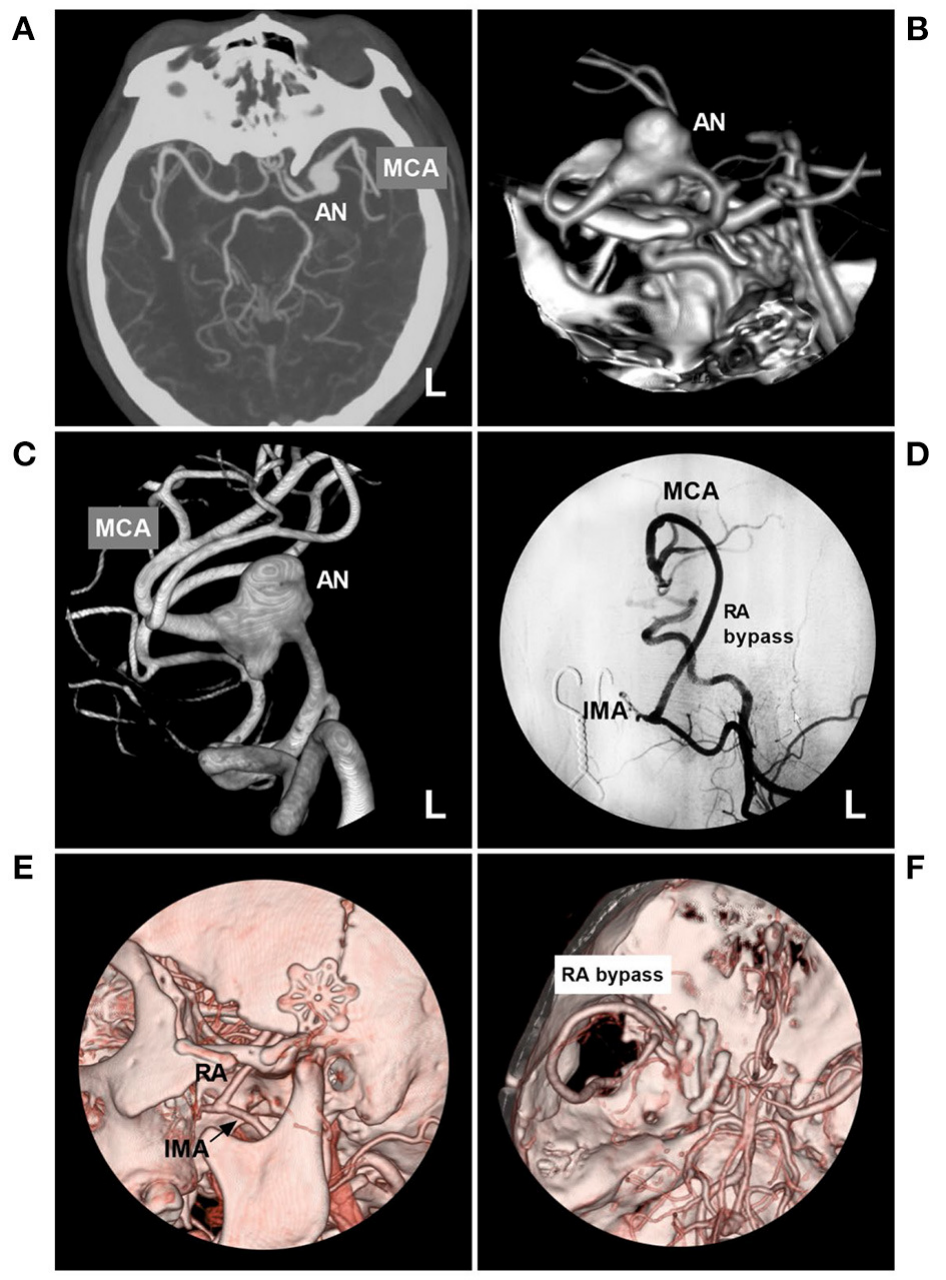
PAO with the assistance of high-flow bypass in a circumferential AN of the MCA trunk. (A,B) Maximum-intensity projection (A) and three-dimensional reconstruction (B) of CTA showed a left circumferential AN of the MCA trunk (AN). (C) Three-dimensional DSA of the left ICA clearly showed the AN. (D) Left DSA showed that high-flow bypass was performed between the IMA and MCA beyond the AN with the RA graft. (E,F) Follow-up CTA showed the bypass. (C) the RA was anastomosed to the IMA trunk (arrow). (F) the RA was anastomosed to the MCA, and the AN was trapped by multiple clips. AN, aneurysm; CTA, computed tomography angiography; DSA, digital subtraction angiography; MCA, middle cerebral artery; ICA, internal carotid artery; IMA, internal maxillary artery; L, left; RA, radial artery; PAO, parent artery occlusion.
